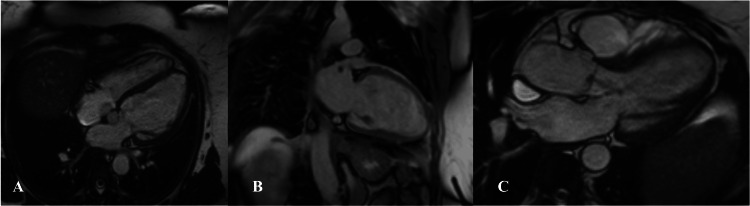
CMR demonstrating borderline left ventricular function, ventricular dilatation, and aortic regurgitation (A) Axial cine CMR demonstrating dilated ventricles with preserved myocardial contours, consistent with borderline left ventricular systolic function. (B) Sagittal cine CMR view showing left ventricular chamber enlargement, supporting the presence of ventricular dilatation associated with borderline ejection fraction. (C) Cine CMR demonstrating a visible aortic regurgitation jet during diastole, indicating the presence of aortic regurgitation without quantitative assessment. CMR: cardiac magnetic resonance imaging
